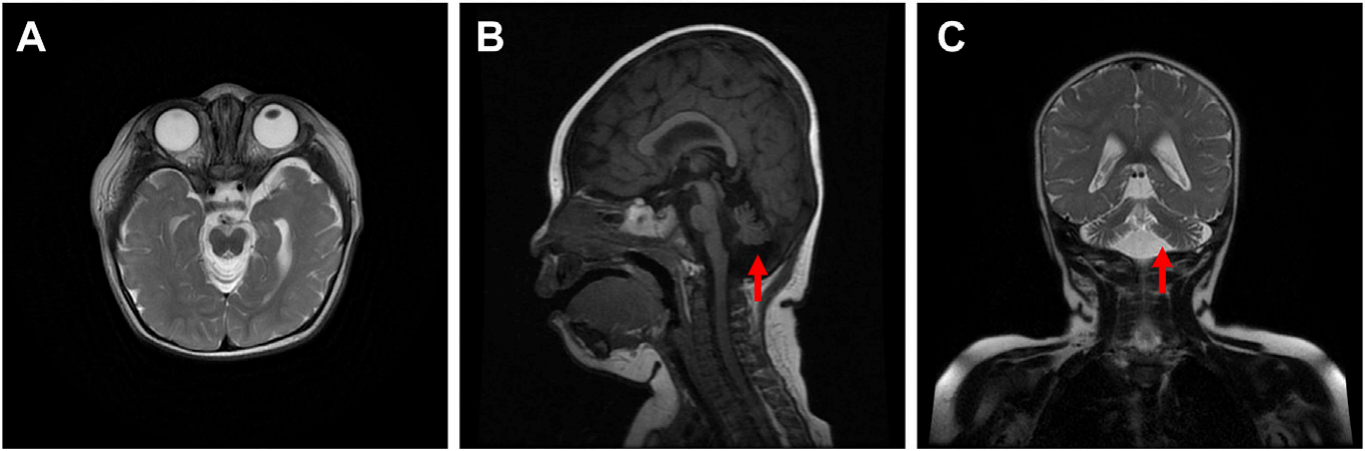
Axial (A), sagittal (B), and coronal (C) brain magnetic resonance images of the proband.
